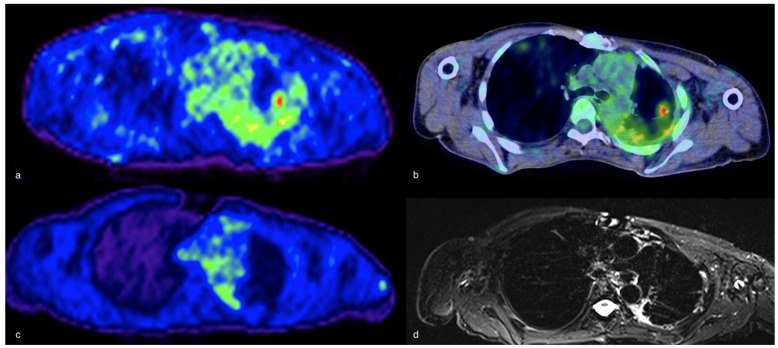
^18^F-FDG-PET (a) and fused axial ^18^F-FDG-PET/CT (b) demonstrating a pulmonary infection with high metabolic activity in the left upper lobe which then decreased after treatment with antibiotics at ^18^F-FDG-PET/MR (fused axial image in (c) and axial STIR in (d).
